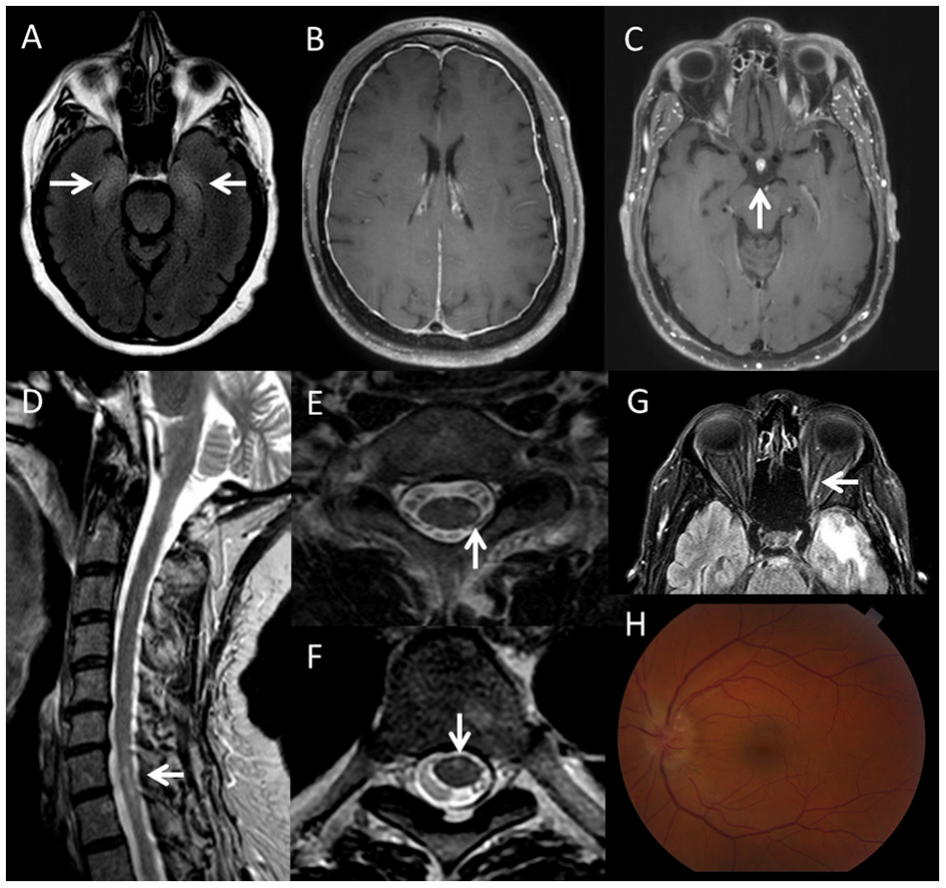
Imaging findings in patients with neurological immune related adverse events triggered by immune checkpoint inhibitors. (A) Axial brain MRI, fluid attenuation inversion recovery (FLAIR) sequence, showing bilateral T2-hyperintensity of the medial temporal lobes (right more than left), in a patient with limbic encephalitis associated with an unclassified neural-specific antibody, after treatment with nivolumab for melanoma. (B) Axial brain MRI, T1 post-gadolinium sequence showing dural enhancement in a patient with meningitis during treatment with nivolumab for sarcoma. (C) Axial brain MRI, T1 post-gadolinium sequence, showing enhancement of the hypophysis in a patient with hypophysitis after atezolizumab treatment. (D) Sagital T2 cervical spine MRI, (E) axial T2 cervical spine MRI and (F) axial T2 thoracic spine of a patient who developed short-segment (multiple sclerosis-like) spinal cord lesions (left lateral cord at C7–T1 and ventral cord at T5–T6) after treatment with nivolumab and ipilimumab for melanoma. (G) Axial FLAIR brain MRI showing T2 hyperintensity of the left optic nerve in the same patient. (H) Optic disc edema in a patient with bilateral optic neuritis triggered by atezolimumab treatment for small cell lung cancer, associated with positive CRMP-5 IgG.
